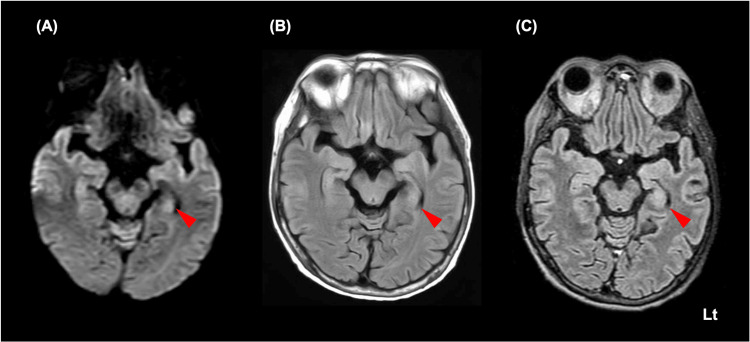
Brain magnetic resonance imaging Although prominent abnormal findings are not visible, there might be high intensity in the left mesial temporal region (red arrowhead) in the diffusion-weighted imaging (A), T2-weighted FLAIR imaging (B), and contrast-enhanced 3D FLAIR imaging (C). FLAIR, fluid-attenuated inversion recovery
